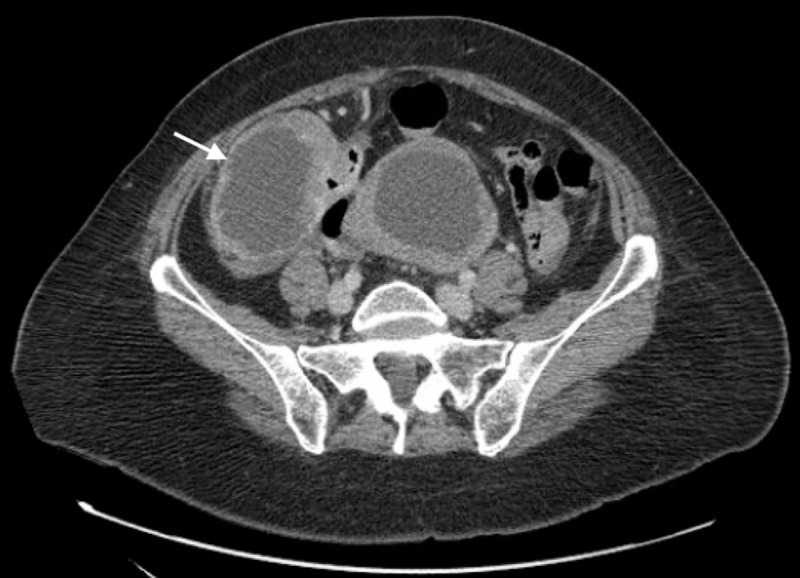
Computed tomography angiogram (CTA) showing a large pelvic, exophytic, necrotic mass with hypervascularity adjacent to the uterine fundus with invasion of the mass into small bowel. Hyperdense material (consistent with blood products) within the lumen of the bowel can be observed.
